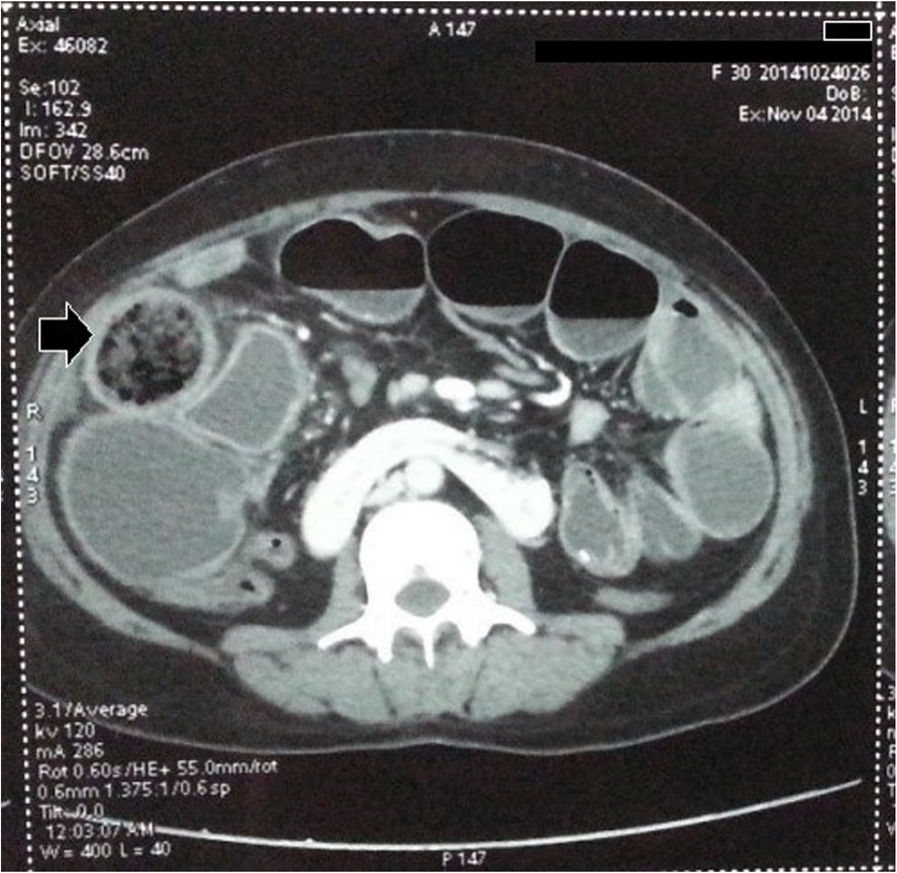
Computed tomography of the abdomen, showing intraluminal gossypiboma (Black arrow)
